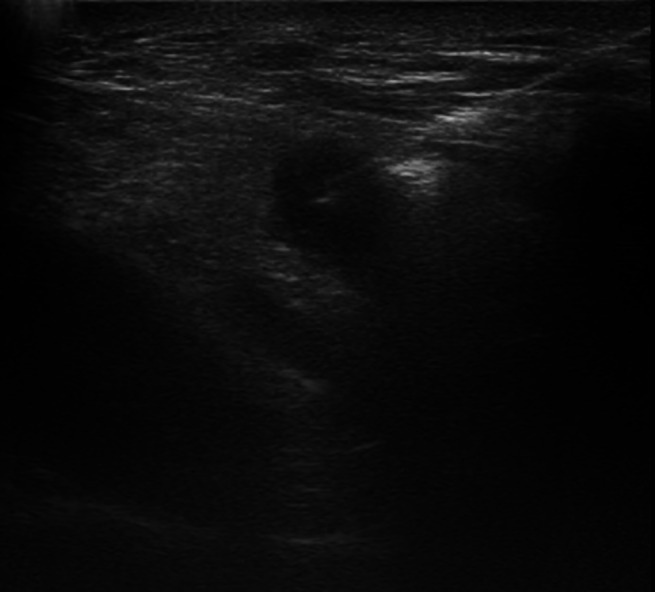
Ultrasound‐guided placement of a pre‐surgical guidewire in a supraclavicular metastasis.
